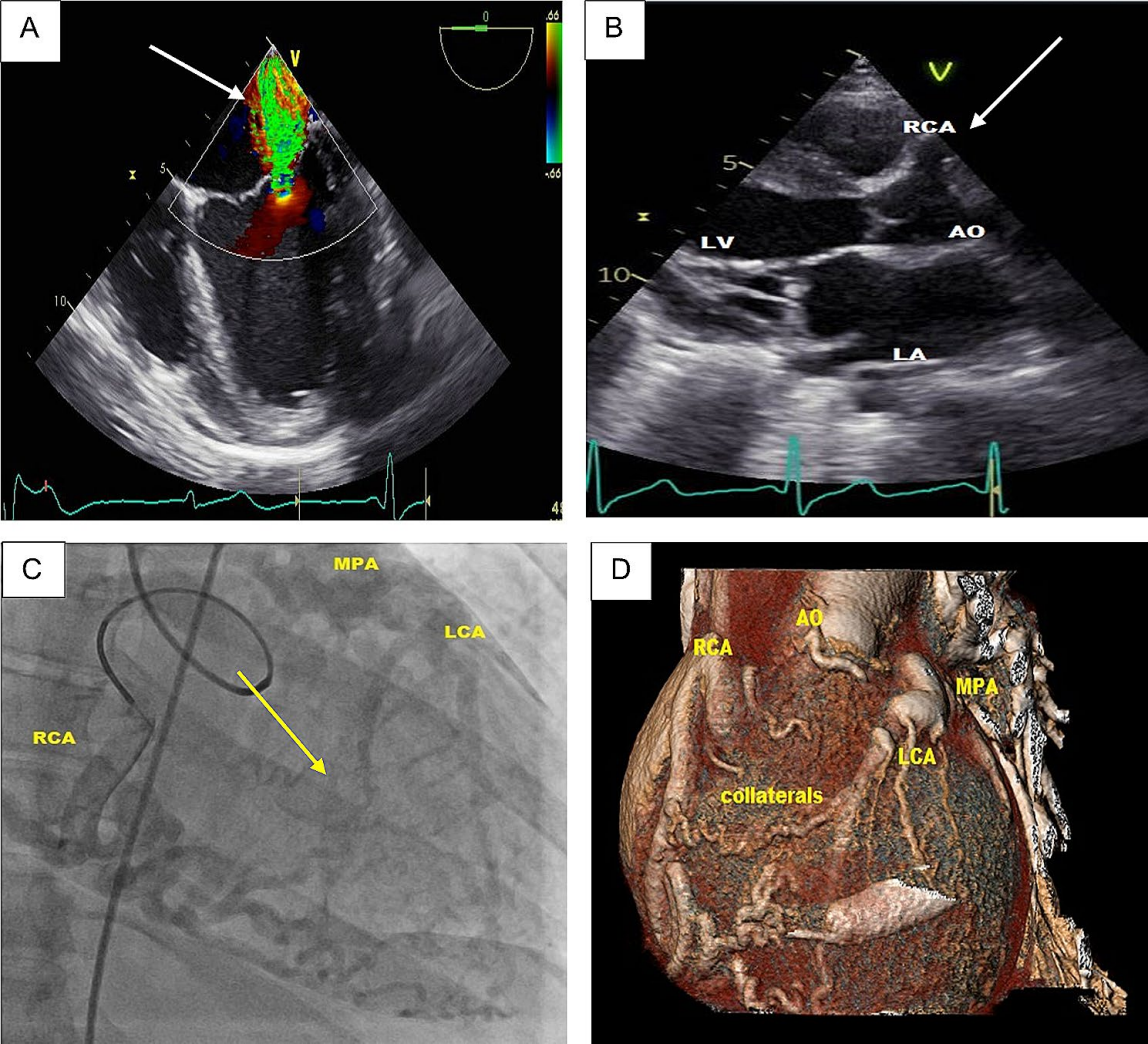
Preoperative diagnostic images. A) Transthoracic echocardiogram showing regurgitation jet (arrow) of the mitral valve. B) Transthoracic echocardiogram (parasternal view) showing the dilated right coronary artery (RCA) ostium (arrow). C) Coronary angiogram demonstrating a tortuous dilated RCA giving intercoronary collaterals (arrow) to the left coronary artery (LCA) which drains into the main pulmonary artery (MPA). D) CT scan with three-dimensional reconstruction demonstrating ALCAPA syndrome. AO: Aorta, LA: left atrium, LV: left ventricle, RV: right ventricle, RCA: right coronary artery, LCA: left coronary artery, MPA: main pulmonary artery, CT: computer tomography
